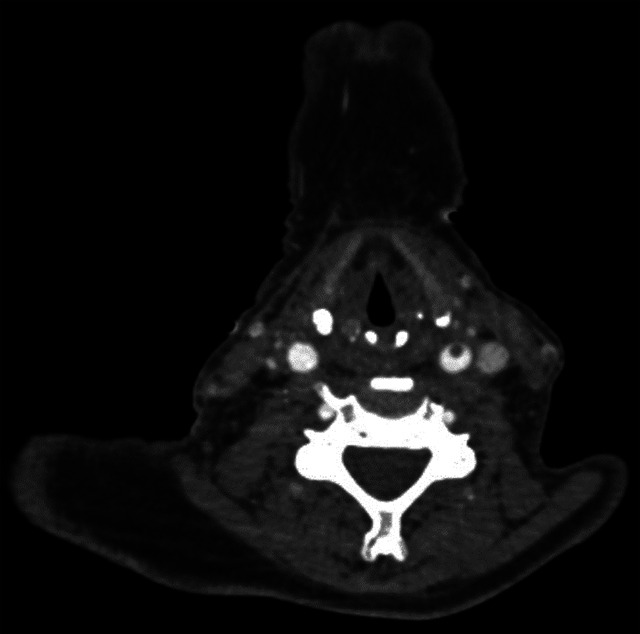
Axial reformatted CTA image also shows the luminal thrombus in the left common carotid artery.
